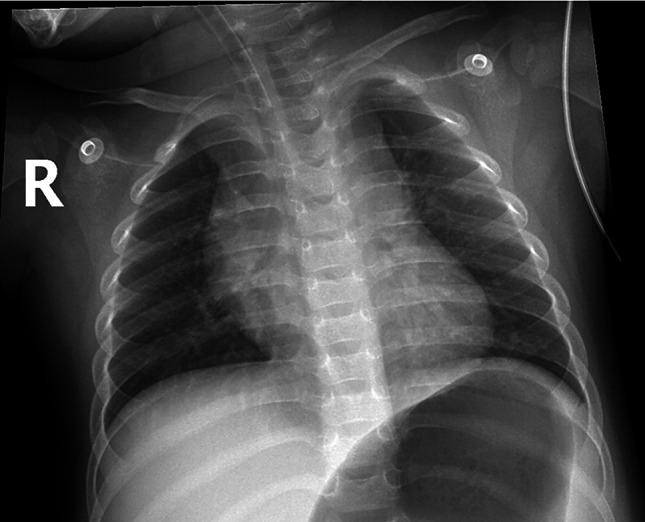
Portable chest radiograph on admission (29/05/2025). Portable anteroposterior chest radiograph demonstrating bilateral perihilar bronchial wall thickening and peribronchial cuffing, suggestive of acute bronchitis or early bronchopneumonia. No evidence of focal consolidation or pleural effusion is noted. The radiopaque silhouette of an endotracheal tube is present, with its tip approximately 2 cm above the carina—consistent with appropriate placement for mechanical ventilation
